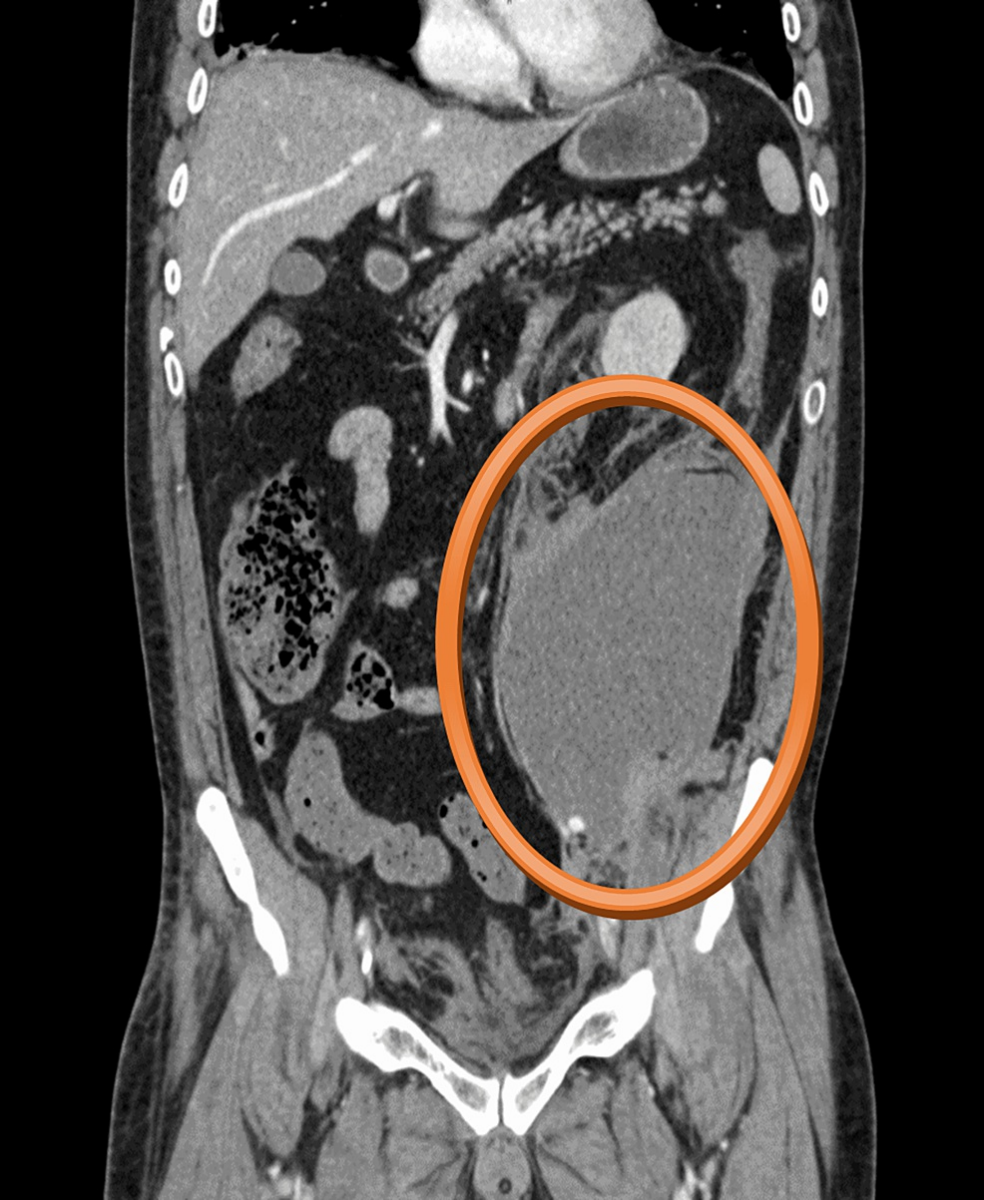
CT scan - coronal plane The hematoma in the left retroperitoneum was of size 170 x 125 x 120 mm, attached to the left musculus psoas major, with present active bleeding
